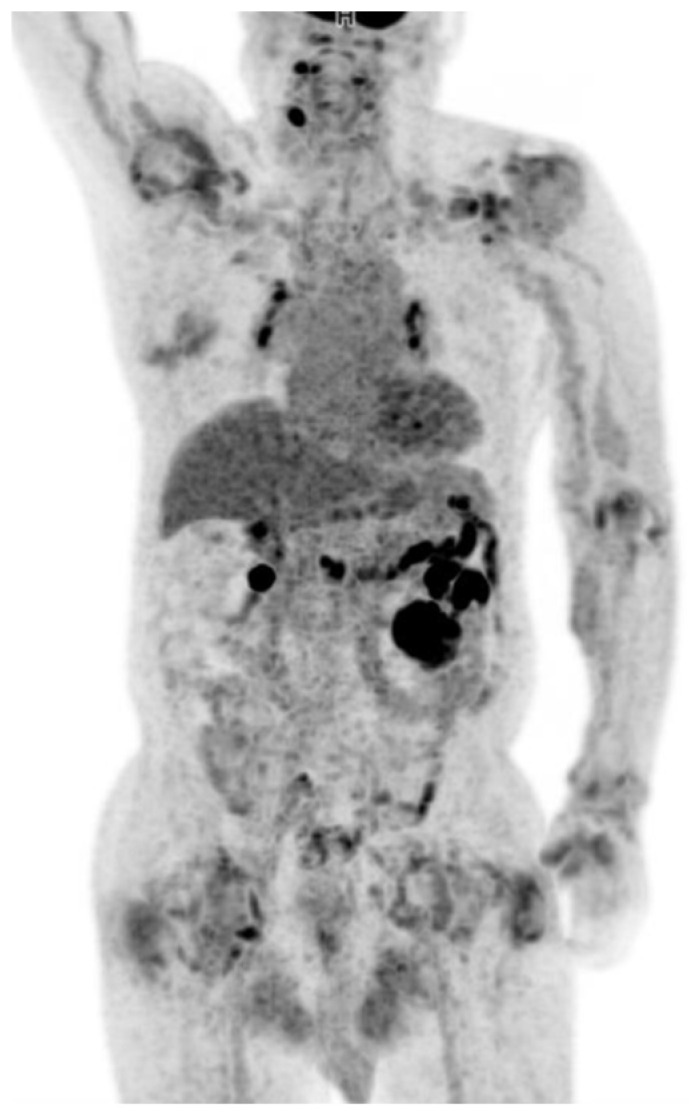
Maximum intensity projection of ^18^F-FDG PET-CT demonstrated increased metabolic activity in the shoulders, left elbow, left wrist, left hand and hips.
